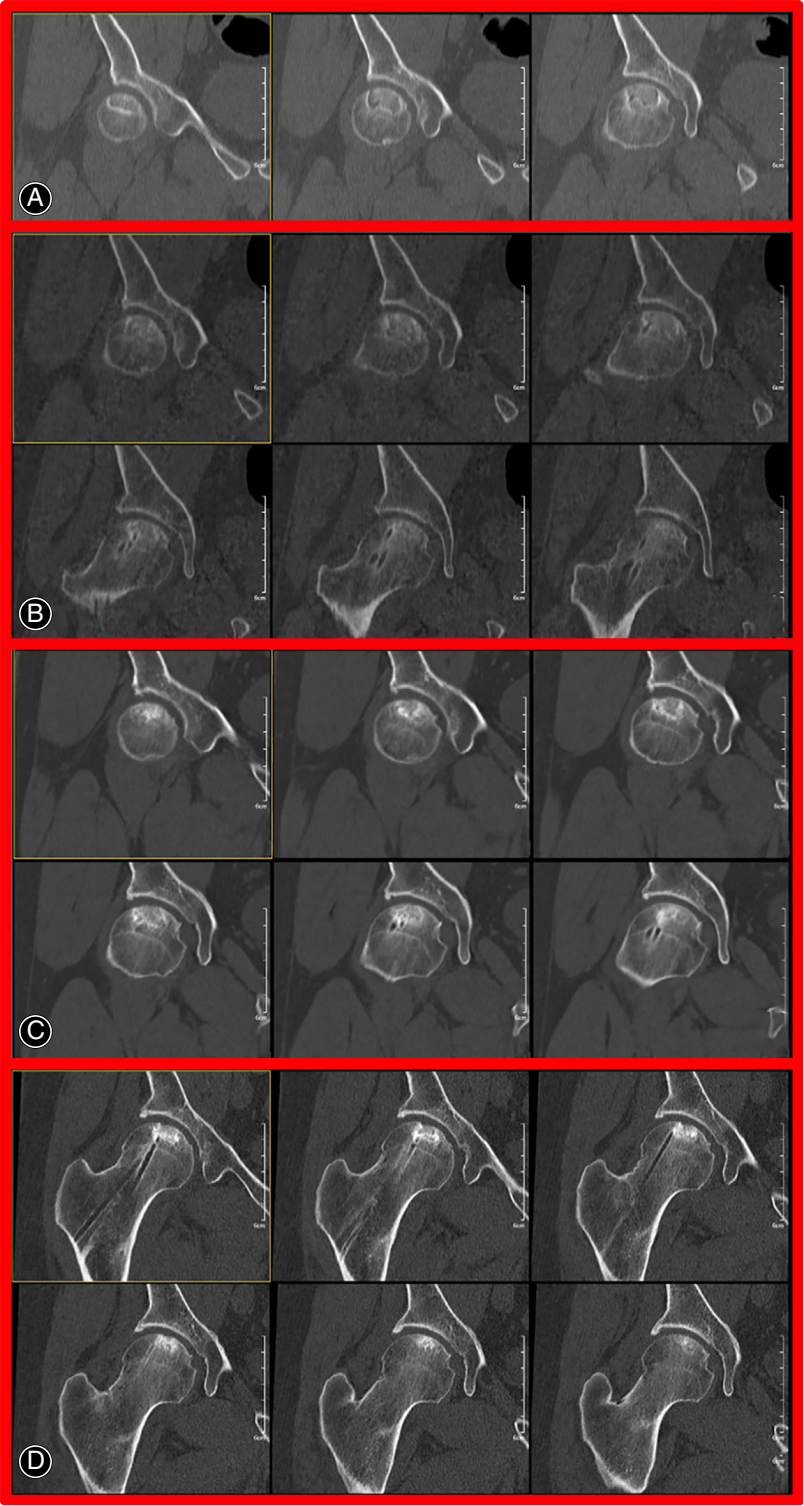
CT of osteonecrosis of right femoral head pre‐ and post‐operation. (A) Obvious necrotic area and bone resorption could be seen pre‐operation. (B) The sclerotic zone gradually disappeared at 16 months. (C) No collapse of the femoral head occurred and obvious hardening in the necrotic area was seen. (D) The outline of the femoral head remained intact at 7 years.
